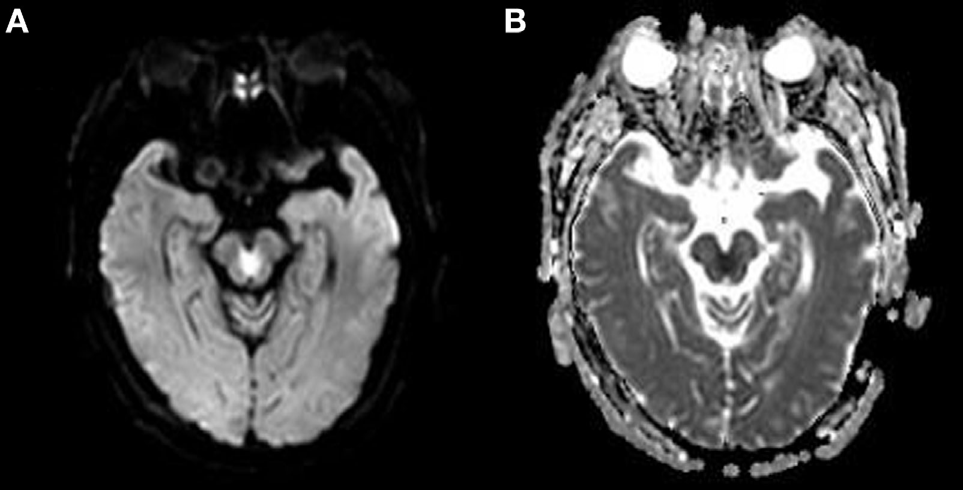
(A) The brain diffusion-weighted image (DWI) of the patient is shown. Note the “heart or V”-shaped lesion showing increased intensity in the tegmentum of the caudal midbrain. (B) The brain apparent diffusion coefficient map MRI (ADC) of the patient is shown. The “heart or V”-shaped lesion shows low intensity on ADC, consistent with acute infarction.
